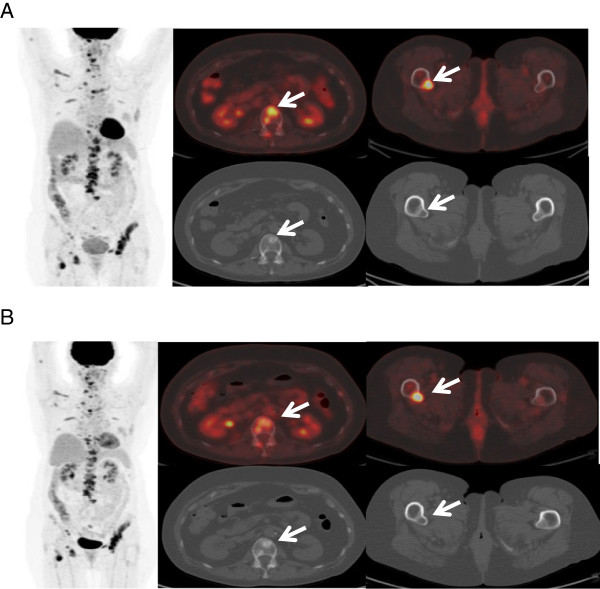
Imaging study with ^18^F-FDG PET/CT. A: ^18^F-FDG PET/CT imaging studies at baseline, before administration of radium-223 (Ra-223) dichloride, B: ^8^F-FDG PET/CT imaging studies after 2 treatments with Ra-223 dichloride but before the third treatment.
